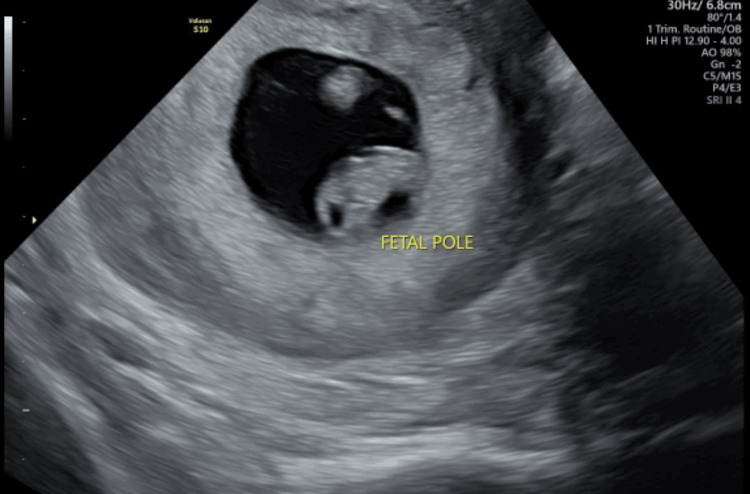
First-trimester transvaginal ultrasound showing singleton fetal pole. This image is included to show consistency in gestational dating by last menstrual period but is not meant to represent the gestational age of the fetus at the time of this case.
